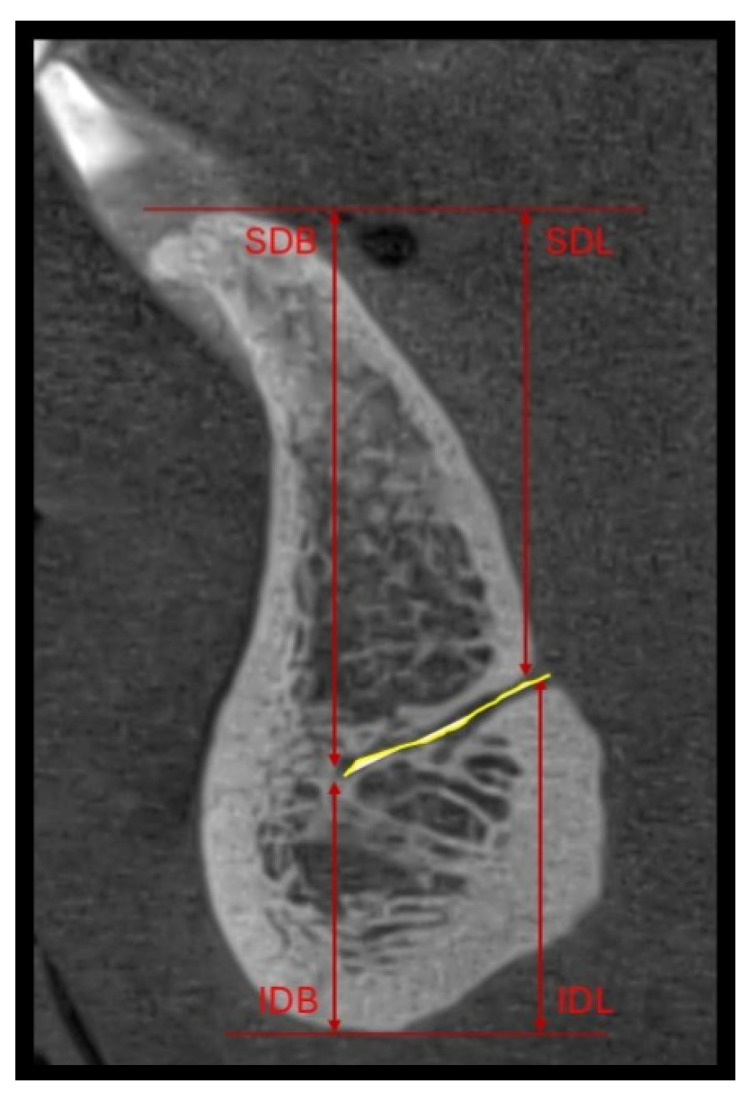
CBCT sagittal cut showing study variable measurements: superior buccal—superior border to the buccal terminal (SDB); superior lingual—superior border to the lingual terminal (SDL); inferior buccal—inferior border to the buccal terminal (IDB), inferior lingual—inferior border to the lingual terminal (IDL); Yellow line—lingual canal.
